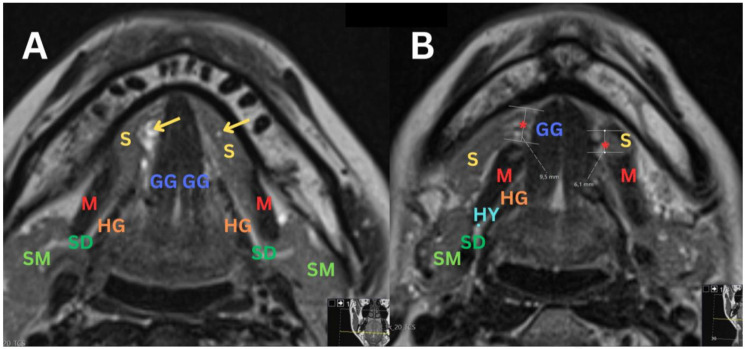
(A) An MRI of the soft tissues of the neck with intravenous contrast administration of T2 TSE Dixon axial sequence, showing a defect in the right mylohyoid muscle (M), accompanied by the protrusion of the right sublingual salivary gland (S) with several small sialoceles indicated by an arrow in the background. Similar but less pronounced changes are observed on the left side, where there is a minor protrusion of the left sublingual salivary gland (S) with small sialocele (arrow). (B) A closer view of the defects in the right mylohyoid muscle (M) using the same T2 TSE Dixon axial sequence, highlighting the protrusion of the right sublingual salivary gland (S). Similar but less pronounced changes are observed on the left side, where there is a minor protrusion of the left sublingual salivary gland (S). S—sublingual gland; M—mylohyoid muscle; *—mylohyoid muscle defect; GG—genioglossus muscle; SM—submandibular gland; SD—submandibular duct (also known as Wharton’s duct); HG—hyoglossus muscle; HY—hypoglossal nerve.
